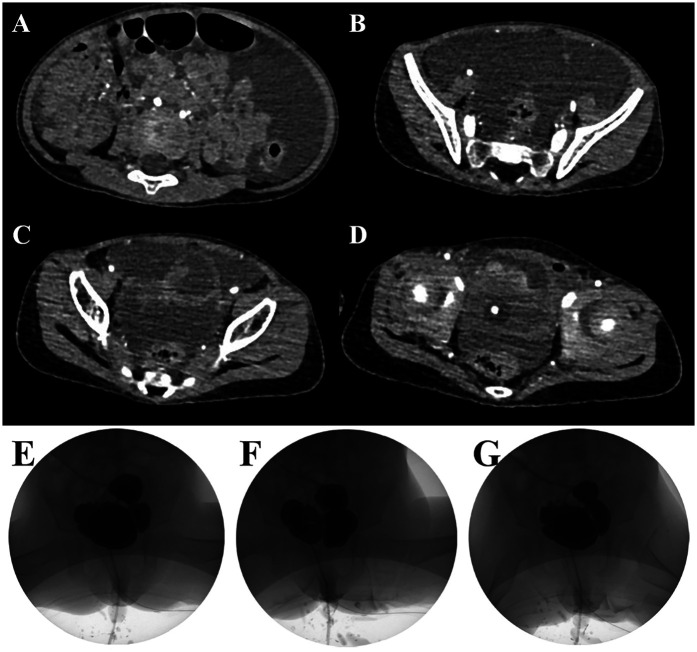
Preoperative abdominal CT scans demonstrating multiple bladder diverticula and ascites in the patient (A–D). Preoperative video urodynamic study showing the bladder filling phase (E), voiding phase (F), and post-void residual phase (G)
